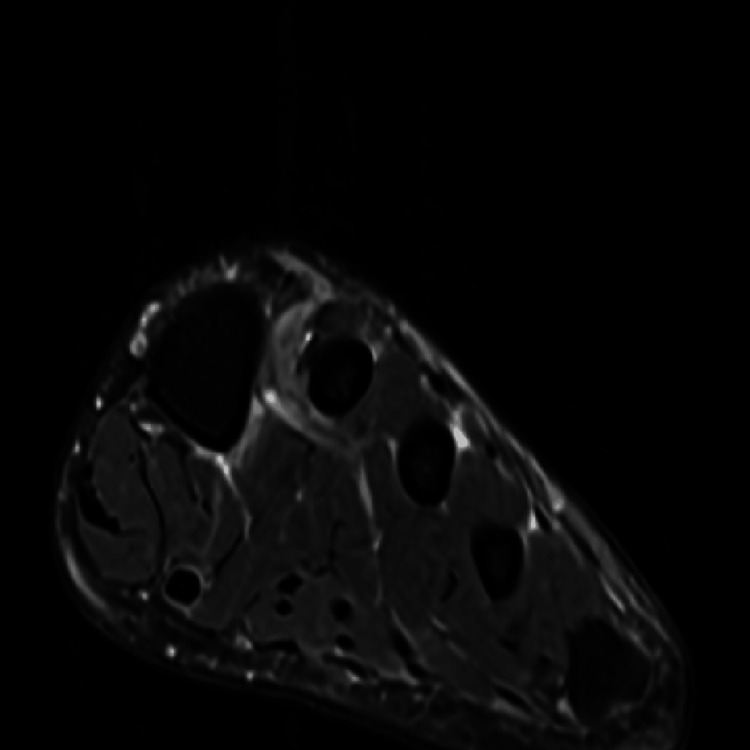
MRI revealing normal vasculature distal to the plantar vein Open venous structures without thrombus inferior to the metatarsals.
